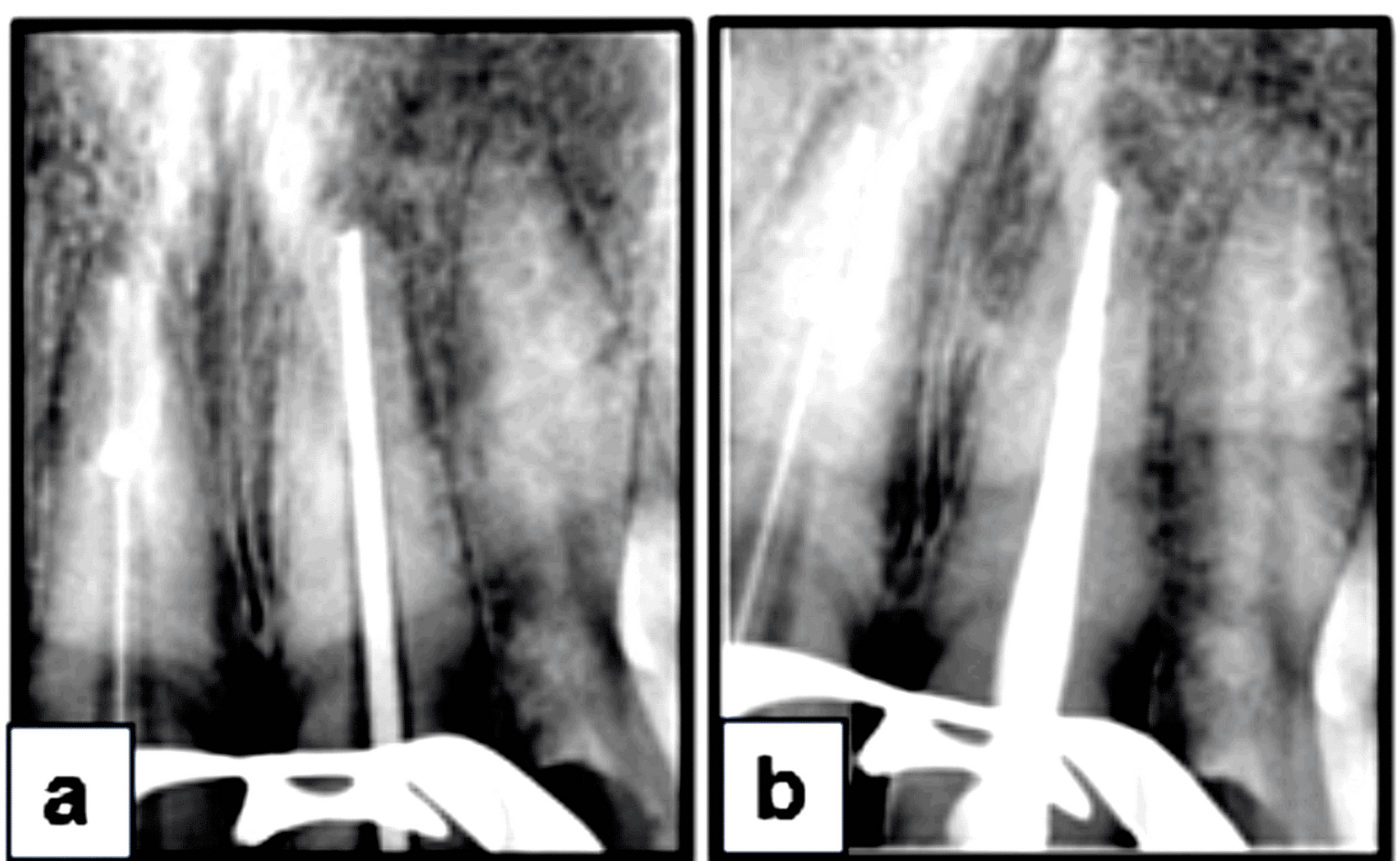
At T=2 weeks: Obturation and post-endodontic restorations. (a) Master cone radiograph. (b) Post-operative radiograph showing obturation in relation to tooth 21.
